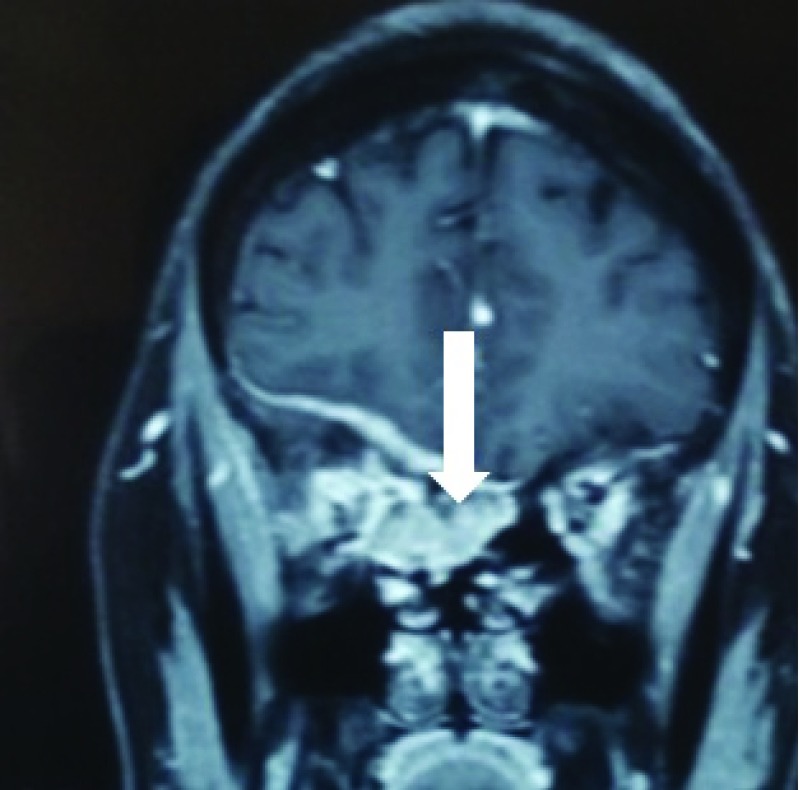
Computed tomography image revealing the presence of invasion of para-nasal sinuses by the lesion.
